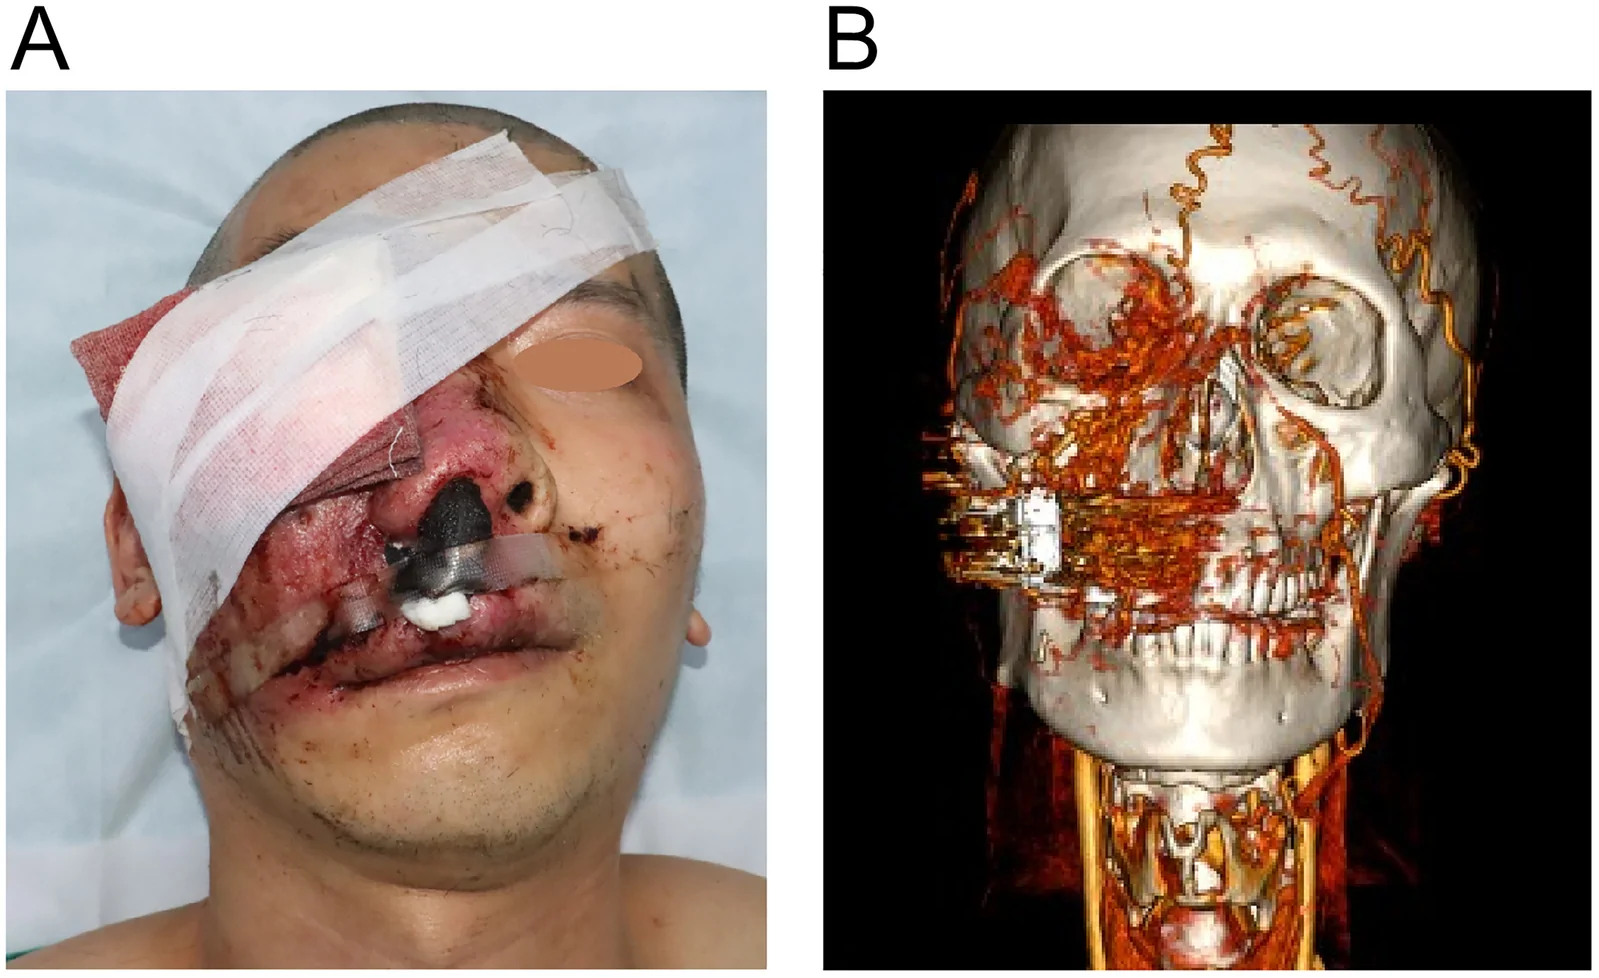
A 33-year-old man with a giant Schobinger stage III/Yakes type IV arteriovenous malformations (AVMs) involving the right orbit and maxillofacial region (Case 1). The patient presented with uncontrolled massive hemorrhage (A). Computed tomography angiography demonstrates that the lesion is supplied predominantly by the right ophthalmic artery arising from the internal carotid artery, with venous drainage into the right internal and external jugular veins (B).
